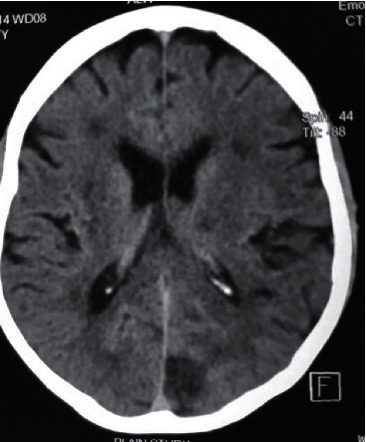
Noncontrast CT scan of the brain showing bilateral watershed infarctions in the fronto-parietal regions and a right-sided occipital infarction.
